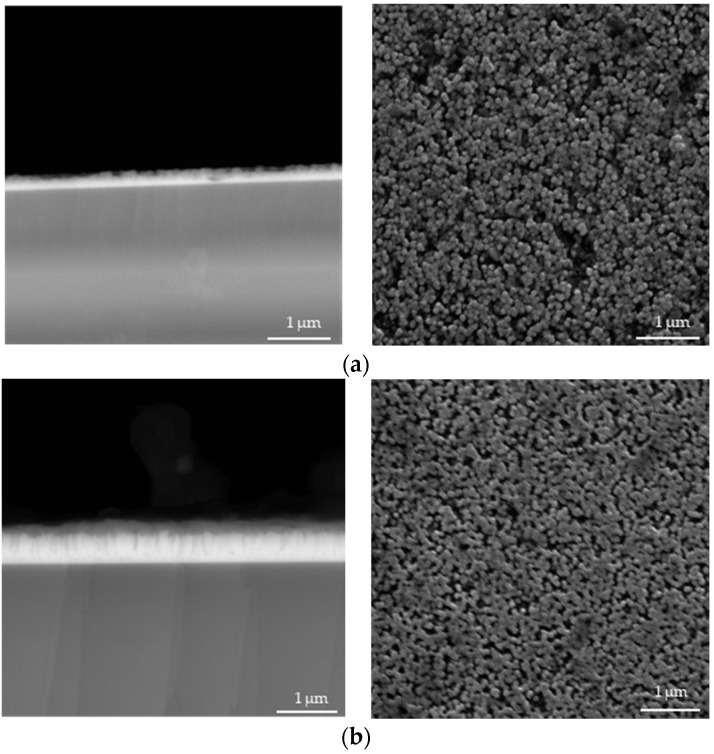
SEM images of nanorods obtained with 1 min of ultrasound and dried for 1.5 h (a) and 2.0 h (b) (left: cross-section image; right: surface image).
